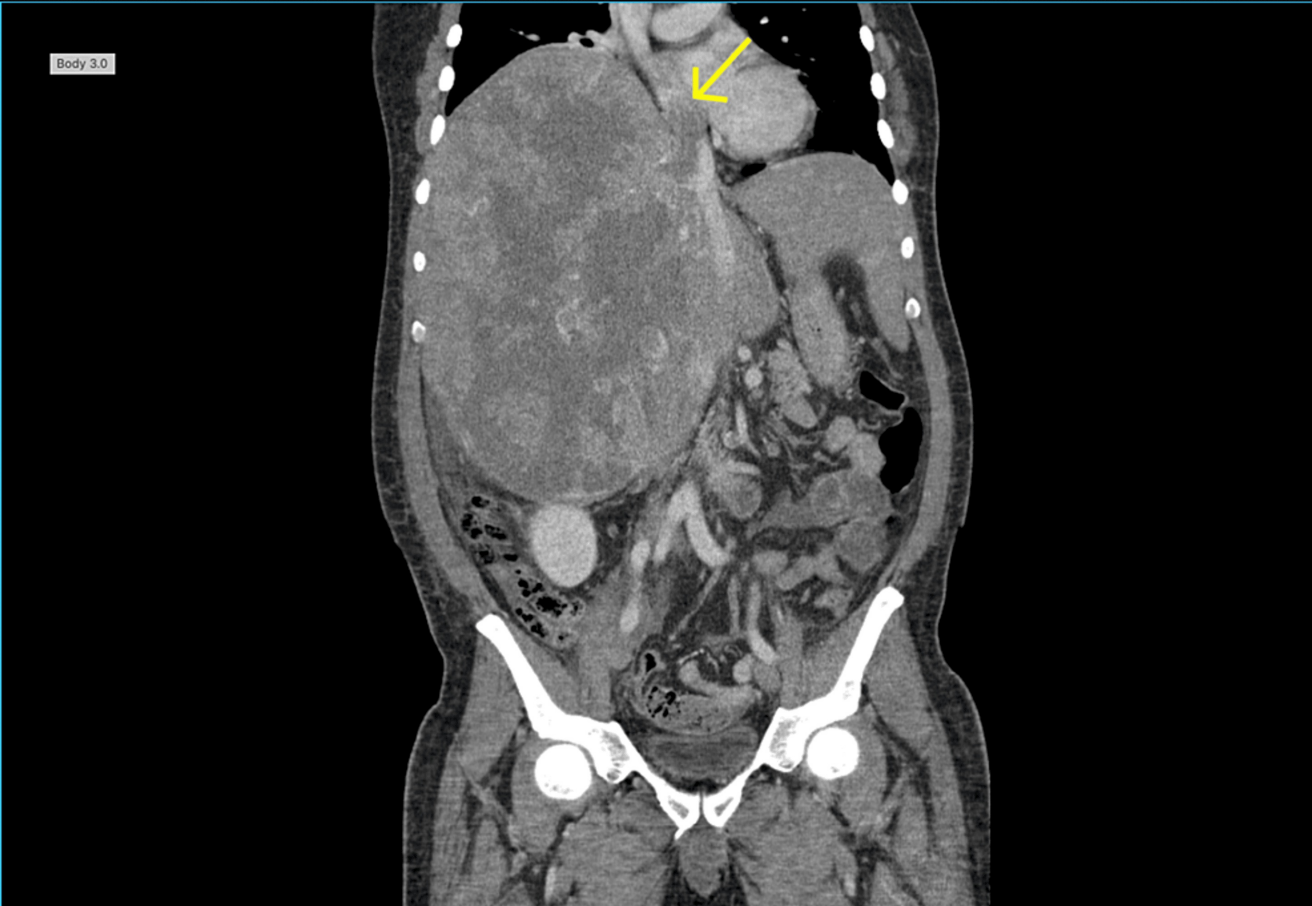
Coronal view of the repeat CT chest and abdomen with contrast Displayed is the extension of the tumor to the IVC and the RA (yellow arrow). IVC: Inferior vena cava, RA: Right atrium
